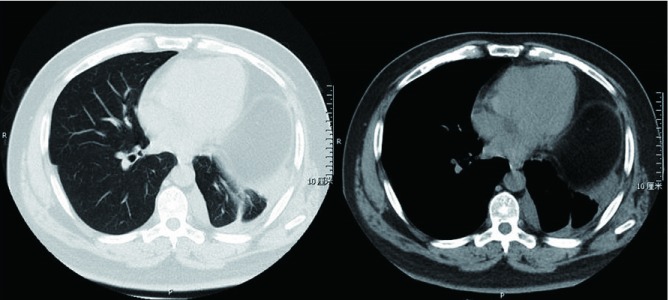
病例1术后6月随访胸部CT Postoperative chest CT image of case No.1 at 6-month follow up
